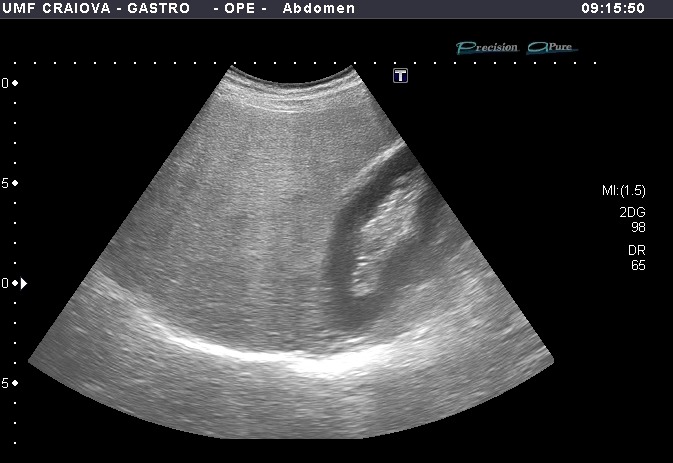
B-mode ultrasound showing hyperechoic liver comparing with the kidney parenchyma and posterior attenuation of the deep liver parenchyma in the context of hepatic steatosis
